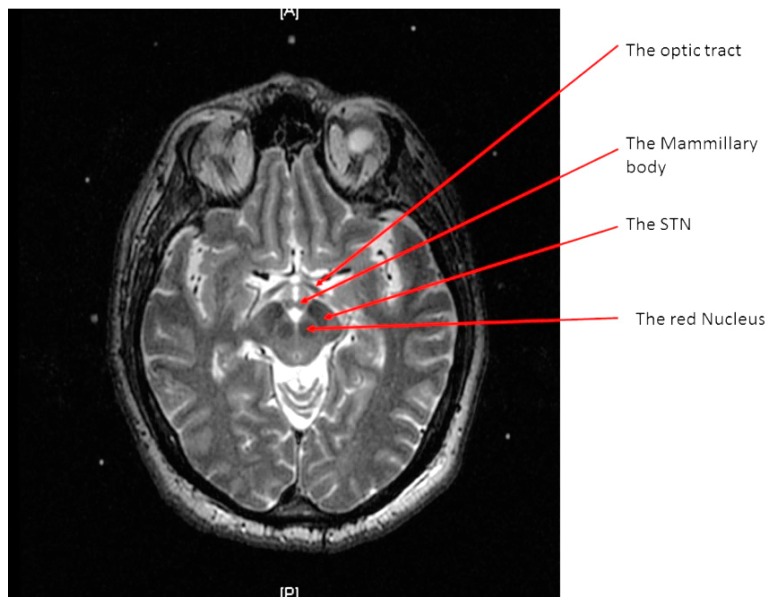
An axial T2 weighted magnetic resonance image (MRI) at the level of the midbrain showing the two subthalamic nuclei (STN).
